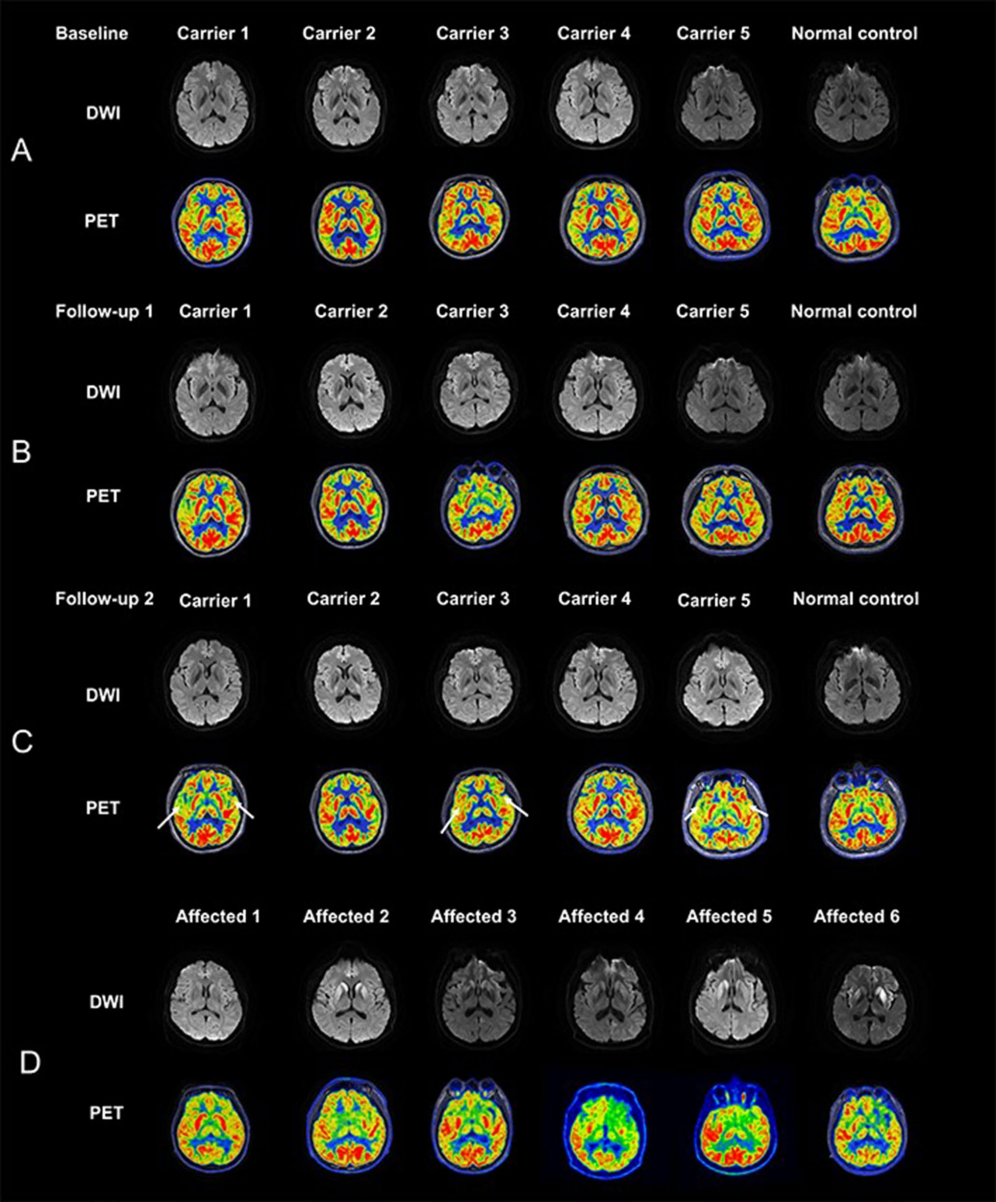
DWI and PET images of asymptomatic PRNP *G114V* carriers and affected gCJD patients. A At baseline, DWI and PET images of five asymptomatic *PRNP* carriers and one normal control showed no abnormality. B At 2-year follow-up, DWI and PET images of five asymptomatic *PRNP* carriers and one normal control showed no abnormality. C At 4-year follow-up, mild hypometabolism was found on PET in carrier 1,3 and 5. No abnormal signal on DWI were found in all subjects (D). High signals were found in thalamus, basal ganglia, or cortex on DWI images in affected patients 2, 5 and 6; Hypometabolism in thalamus, basal ganglia or cortex were found in all six affected gCJD patients
